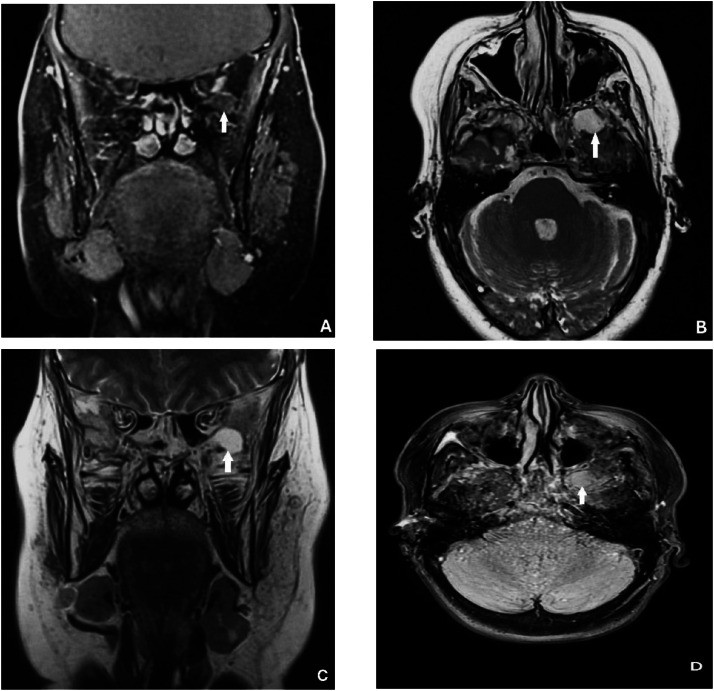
MRI findings. (A) Coronal contrast-enhanced fat-suppressed T1-weighted image showing a well-defined cystic lesion centered in the left greater wing of the sphenoid, appearing hypointense, without internal enhancement (white arrow). (B, C) Axial (B) and coronal (C) T2-weighted images demonstrating marked hyperintensity of the lesion with extension toward the left infratemporal fossa (white arrow). (D) Axial T2-FLAIR image confirming the hyperintense signal of the cystic lesion (white arrow).
